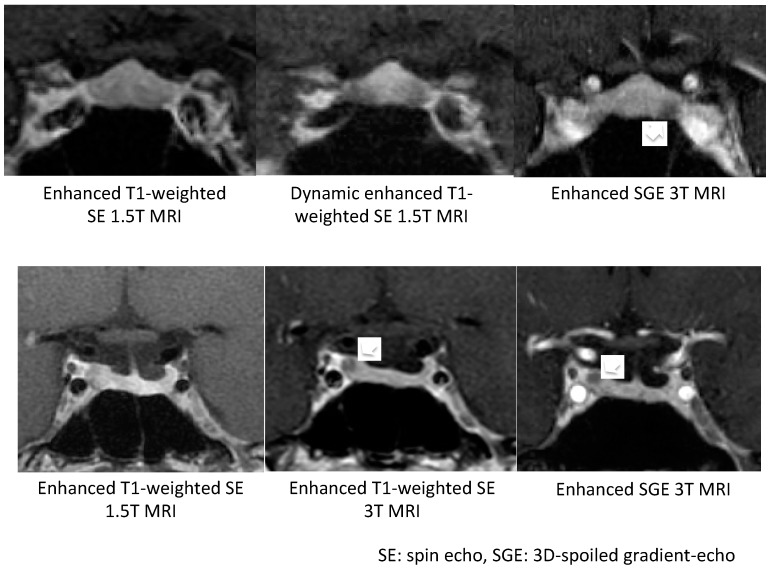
Comparisons of MRI sequences for detecting an adrenocorticotropic hormone (ACTH)-producing micro-tumor (white arrow). The 3D-spoiled gradient-echo (SGE) sequence with 3T-MRI was superior to other conventional MRI sequences in both presented patients.
